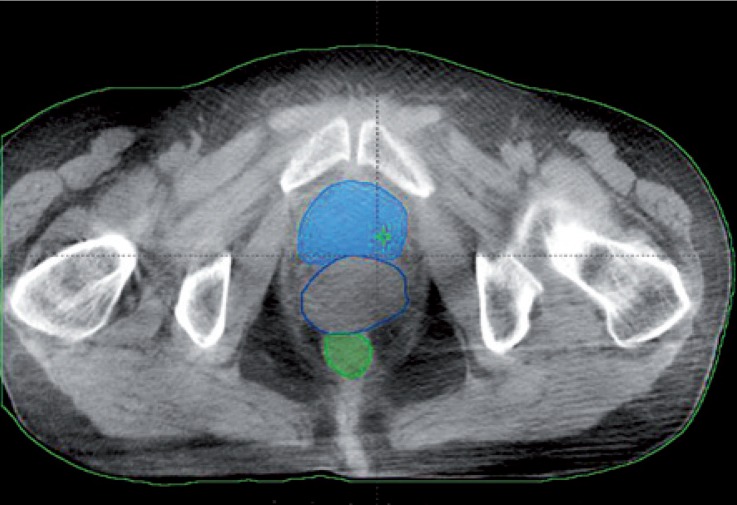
Pelvic organs segmented in cone-beam computed tomography (CBCT) images.
